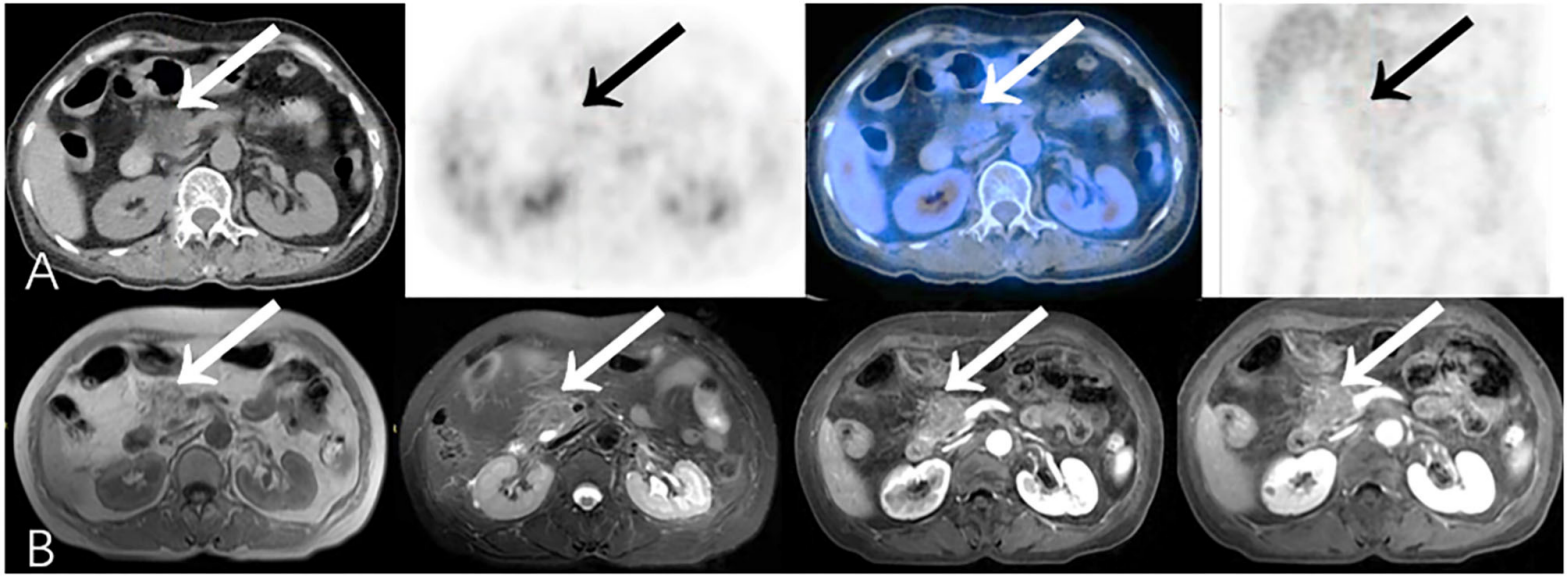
Representative patient on PET/CT (True-Negative) compared to CEMR (False-Positive): A 61-year-old female experienced abdominal pain, nausea and vomiting for 20 days. Serum CA19-9 was normal (12.2 U/ml). (A) PET/CT showed hypodense area without obvious increased focal ^18^F-FDG uptake (SUVmax 1.8) in head of pancreas, which was considered as benign lesion. (B) Lesion was inhomogeneously mildly enhanced on CEMR imaging, presumed more likely to be malignancy. Patient refused to undergo biopsy, and improved after symptomatic treatments. During long-term clinical follow-up (>16 months), patient had no complaints of special discomfort.
